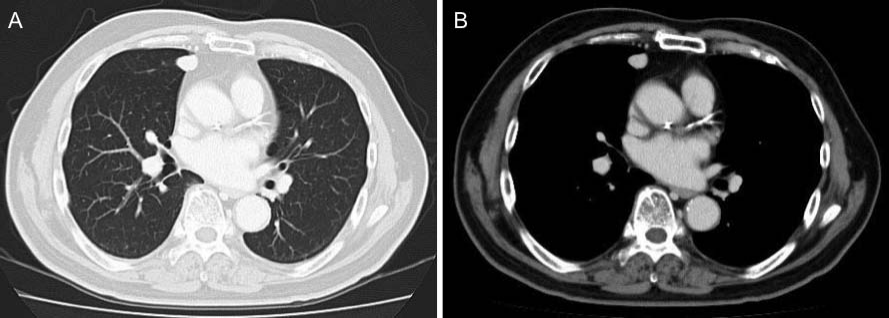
CT findings of solitary pulmonary metastasis. A-B, After pelvic evisceration, chest CT revealed a small nodular shadow (A, Lung window image; B, Mediastinal window image).
